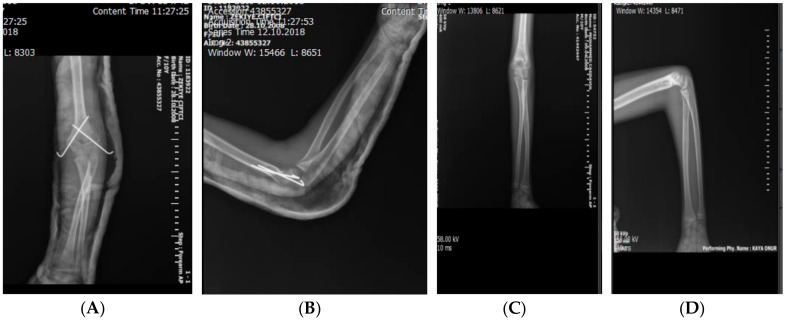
Direct radiographs obtained in the preoperative period and on day 90. (A,B) Postoperative X-ray image showing that the fracture was fixed by two K-wires and (C,D) anteroposterior and lateral views after removal of K-wires three months postoperatively.
